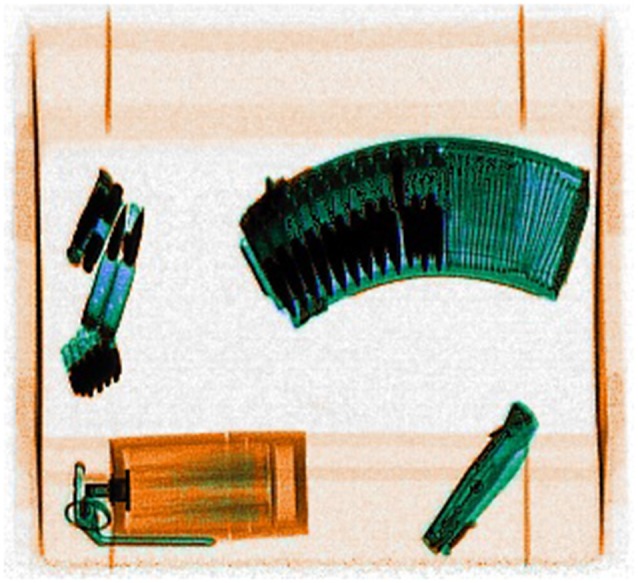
Example threat display presented in the familiarization phase of the x-ray screening task.
